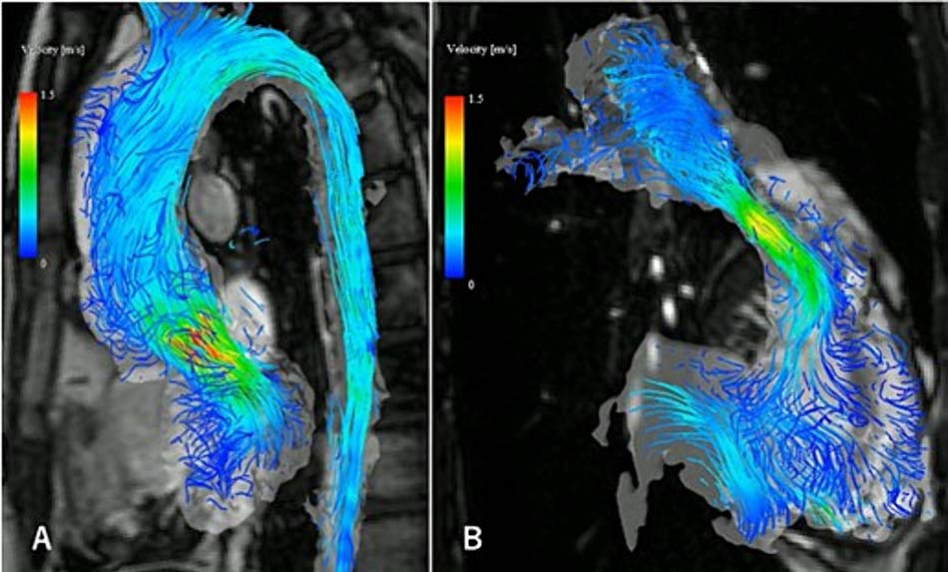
The four-dimensional flow magnetic resonance imaging revealed the presence of mild aortic valve regurgitation (A) and severe pulmonary valve regurgitation (B), with a regurgitant fraction of 34.4%.
